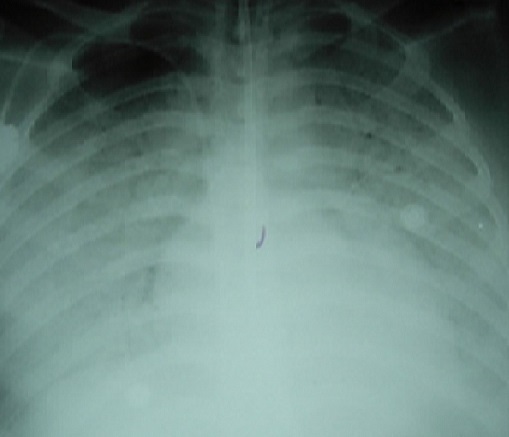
The chest X-ray image revealed bilaterally increased non-homogeneous density on all zones
